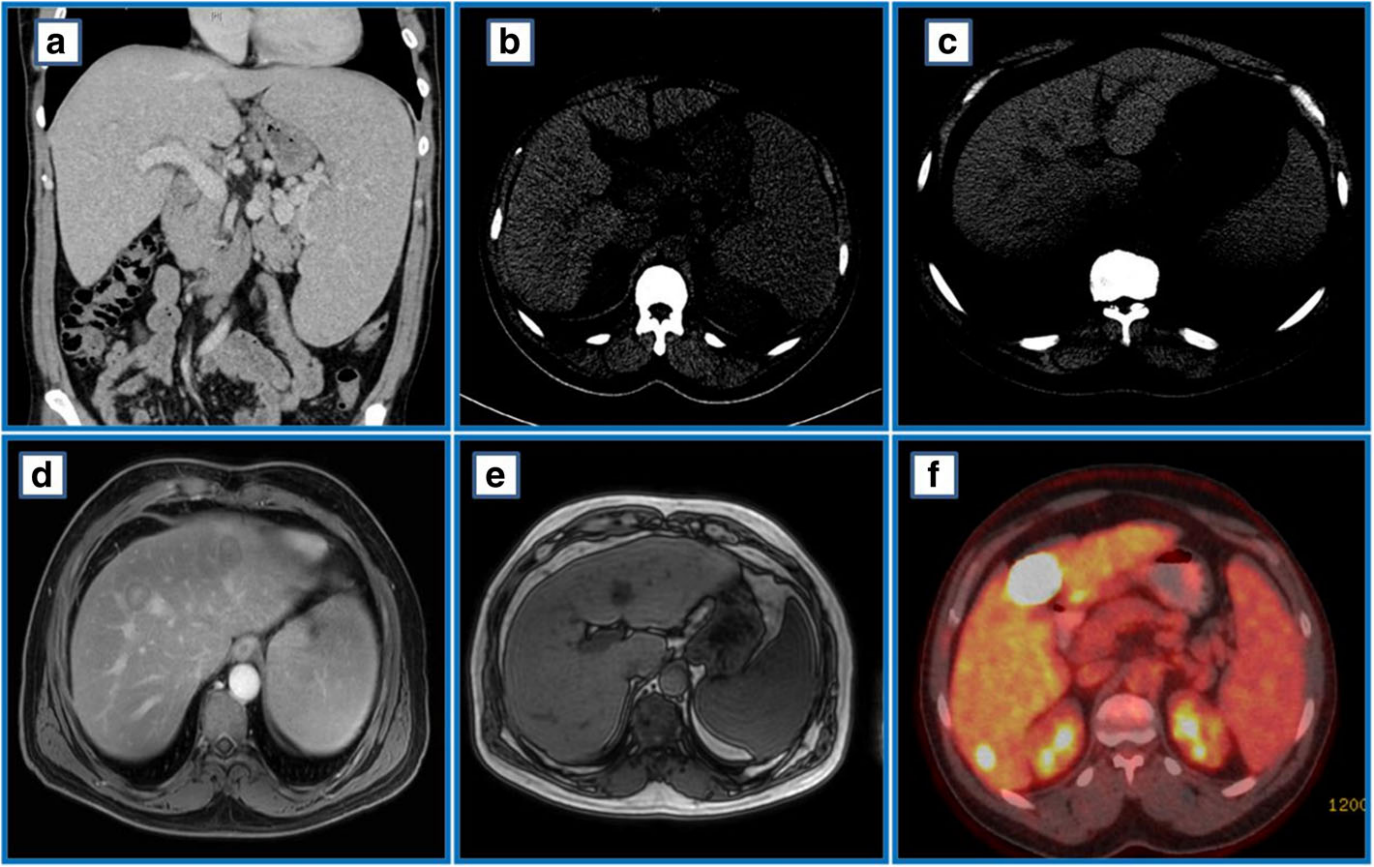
Serial imaging at the time of chronic lymphocytic leukemia diagnosis and at the time of diffuse large B-cell lymphoma diagnosis. a and b Abdominal computed tomography scan in July 2012 showing hepatosplenomegaly with hypodense liver lesions. c Abdominal computed tomography in March 2017 showing hepatomegaly with hypodense liver lesions but no splenomegaly. d and e Abdominal magnetic resonance imaging in April 2017 showing multiple liver lesions appearing slightly high in T2 and low in T1, with restriction in diffusion-weighted images. f Abdominal positron emission tomography/computed tomography in June 2017 showing multiple hypermetabolic liver lesions
